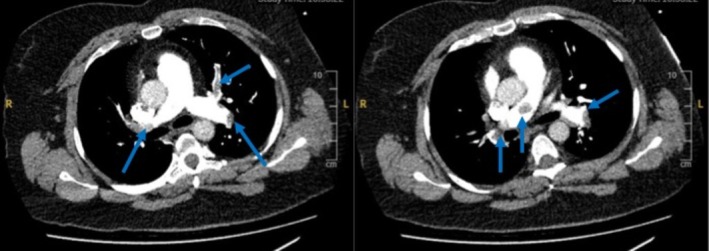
Computed tomography scan of the pulmonary artery showing extensive bilateral thromboembolism in the main and branch pulmonary arteries (blue arrows).
